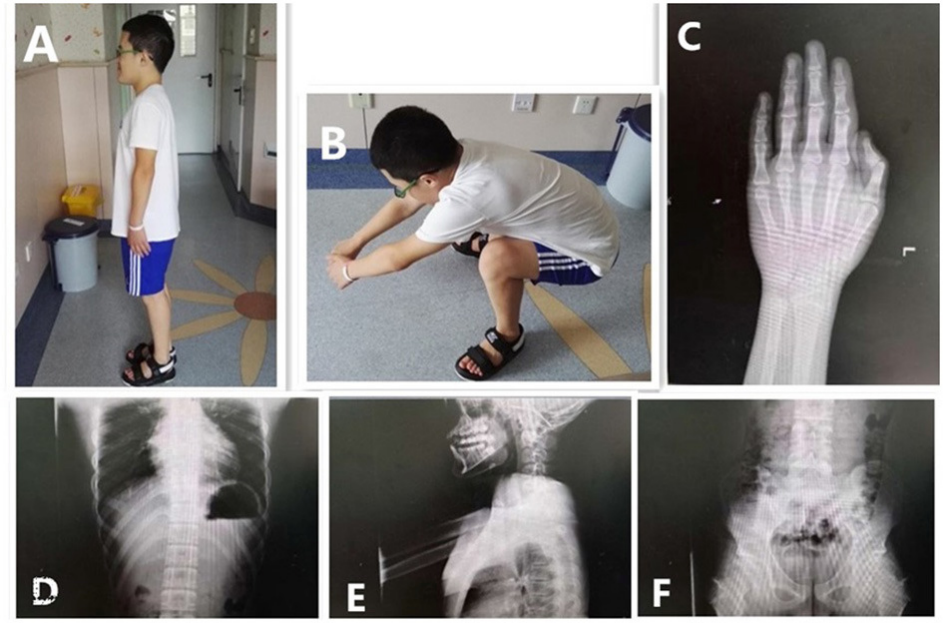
Clinical features and radiographs of the proband at 12.5 years. Special facial features: small ears, prognathism, and short fingers (A). Difficulty in squatting (B). The bone age in the image of the left hand was 16.5 years old; the phalanges were short (C). Anteroposterior and lateral images of the spine showed wide ribs, flat vertebral bodies, large pedicles, and small and hypoplastic iliac wings (D–F).
